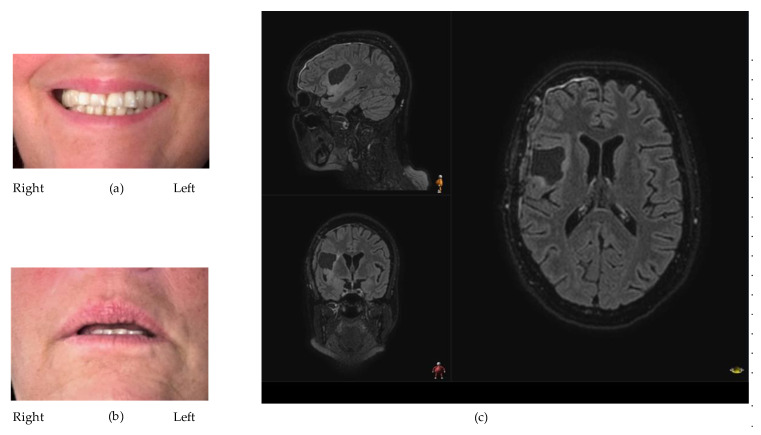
Both the photographs and the MR image (T2 Flair) depict the patient with the most severe facial motor deficit. (a) The patient has been instructed to show as big a smile as possible. A slightly less pronounced left furrow can be noticed. During the execution of the smile, the left side was slightly less responsive. (b) The patient was instructed to relax the face with a slight separation of the lips. A slight lowering of the left corner of the mouth can be noticed. (c) MR image (T2 Flair) of the resection cavity approximately 5 months postop.
